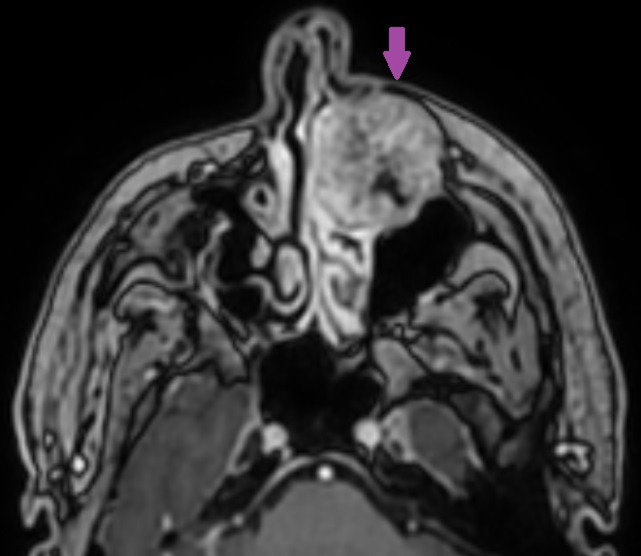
axial contrast-enhanced T1-weighted image showing marked enhancement of the left maxilla lesion (purple arrow)
